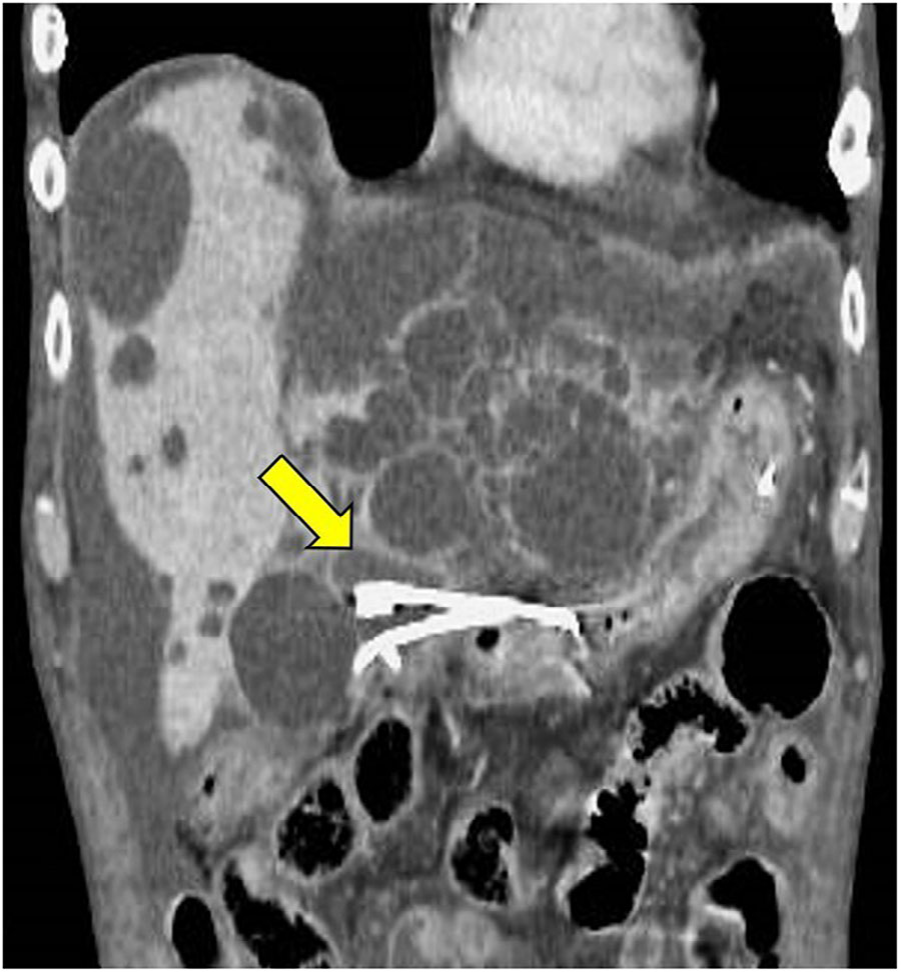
After 11 days of endoscopic ultrasound‐guided drainage, a contrast‐enhanced computed tomography showed almost complete shrinkage of the infectious liver cyst (arrow).
